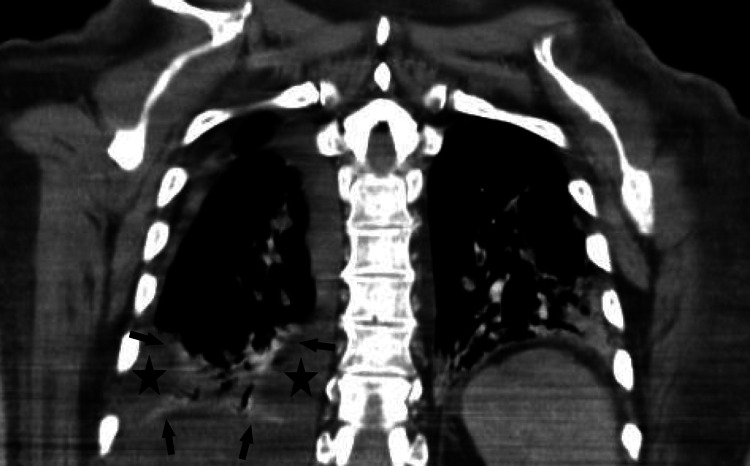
Coronal CT scan of the patient's thorax. The image shows a pleural effusion marked by the stars later confirmed to be a pleural empyema. The arrows indicate the boundary of the right lung.
